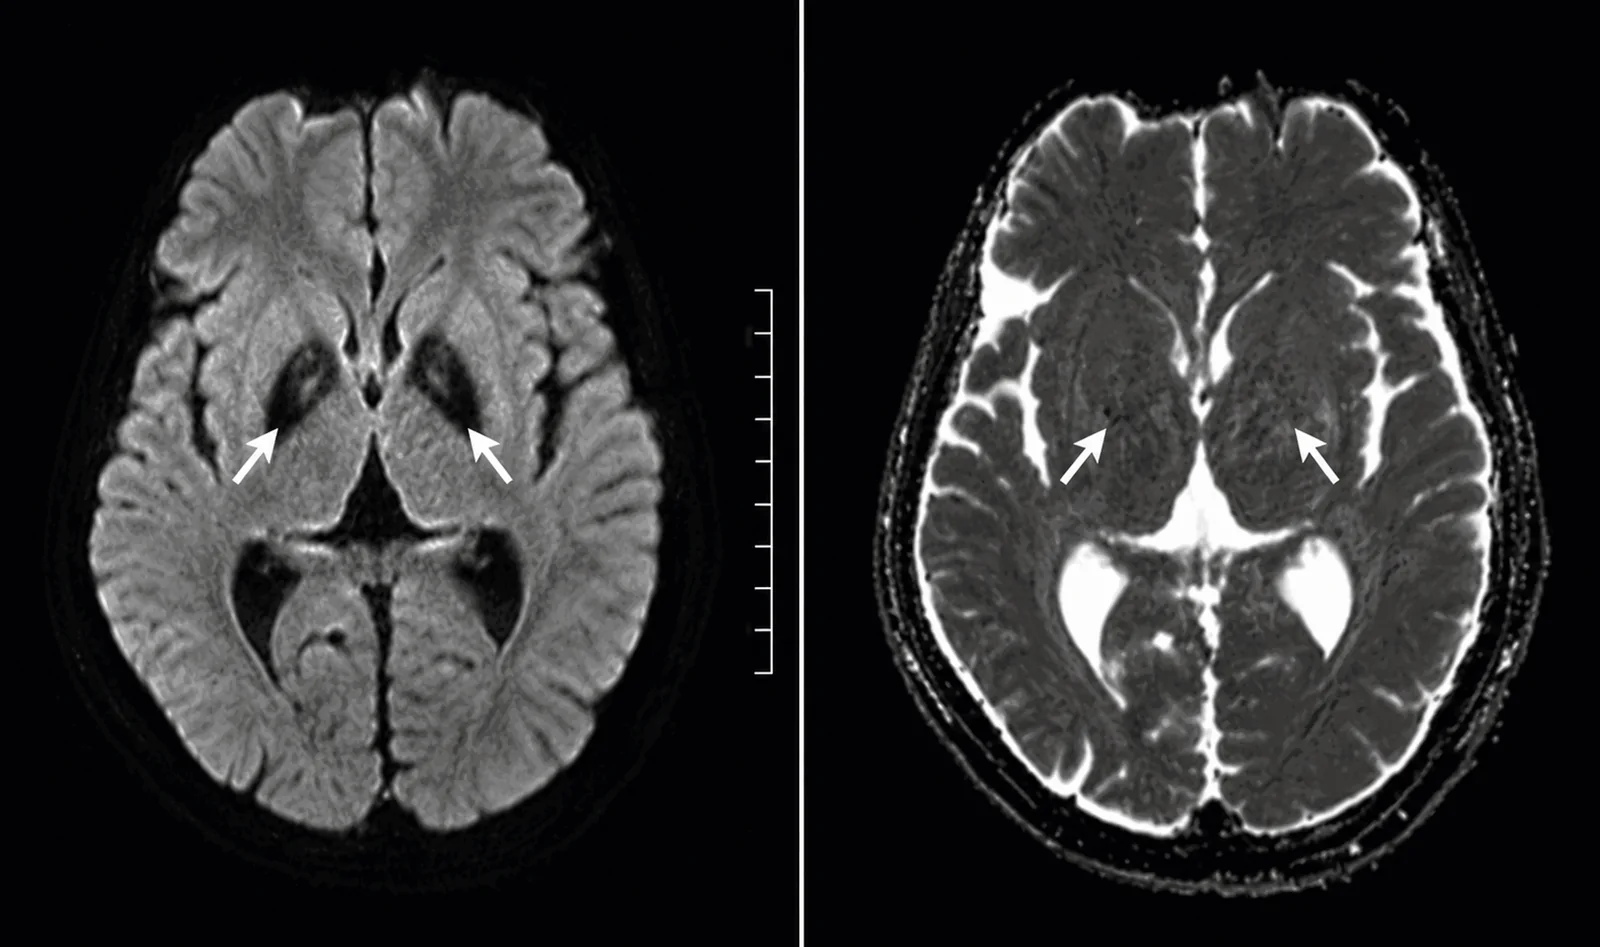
Axial diffusion-weighted imaging (DWI) and corresponding apparent diffusion coefficient (ADC) maps show the bilateral globus pallidus (white arrows) with no evidence of diffusion restriction, effectively excluding acute ischemic pathology.
